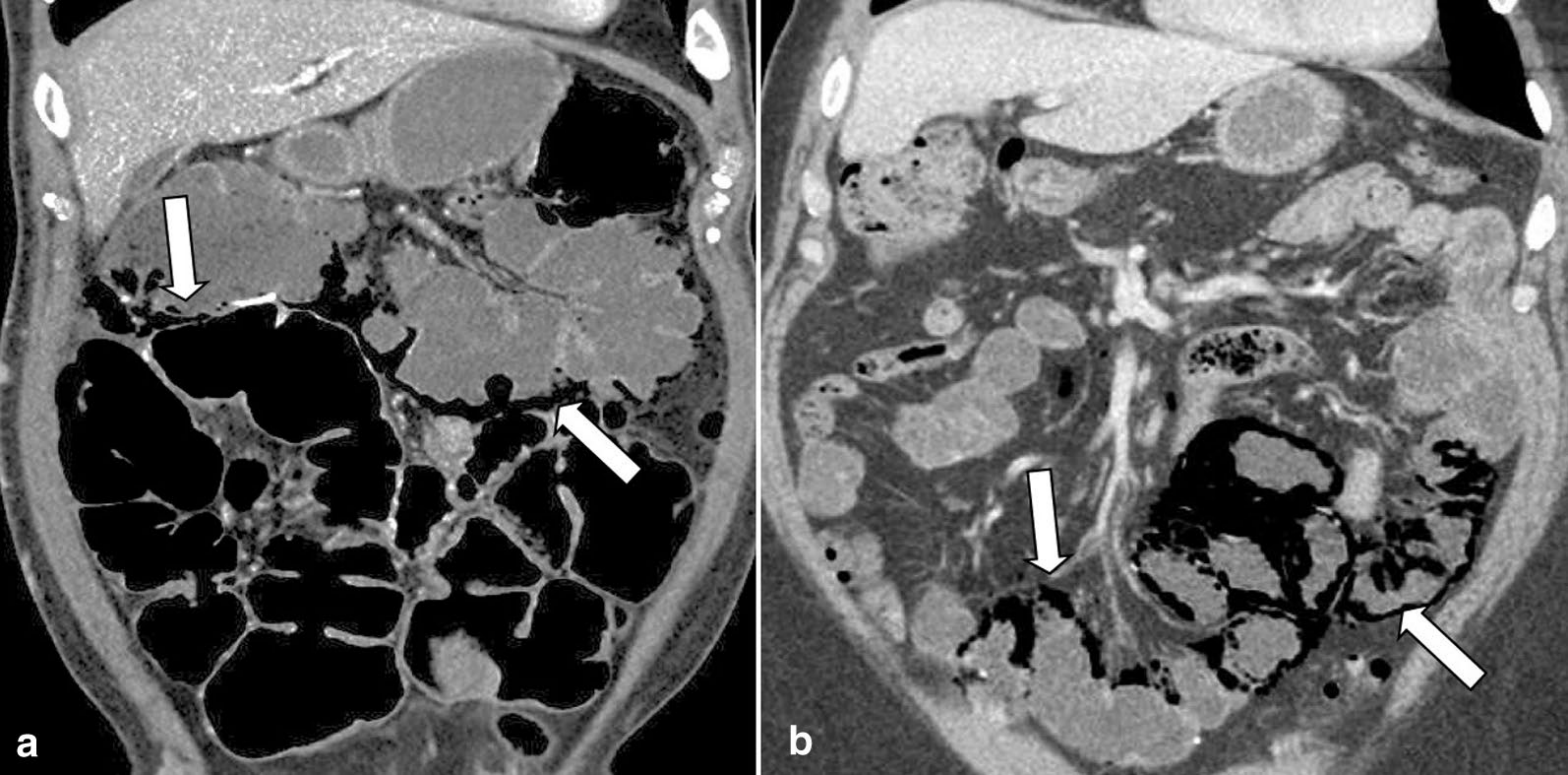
Pneumatosis cystoides intestinalis in two different patients. a A 40 y/o male with Crohn’s disease status post ileocecectomy, on corticosteroids. CT imaging demonstrates benign long-segment colonic pneumatosis cystoides (thick arrows) which resolved on 3 months follow-up examination (not shown). b A 68 y/o male with abdominal pain. CT shows incidental benign pneumatosis of small bowel (thick arrows), but no other findings suggestive of ischemia. Clinical examinatin was benign, and findings proved to be self-limited on follow-up CT
